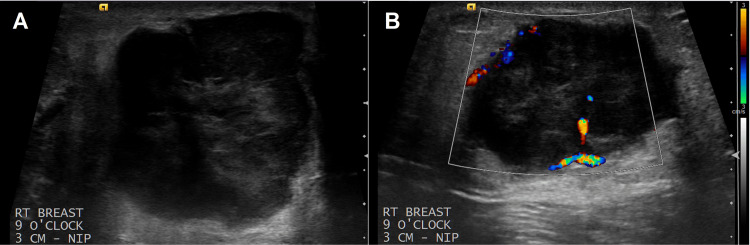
(A) Ultrasonography of the right breast demonstrates a 42 x 32 x 30 mm solid and irregular hypoechoic mass with microlobulated margins at nine o’clock, 3 cm from the nipple. (B) The mass demonstrates increased flow on color Doppler imaging.
